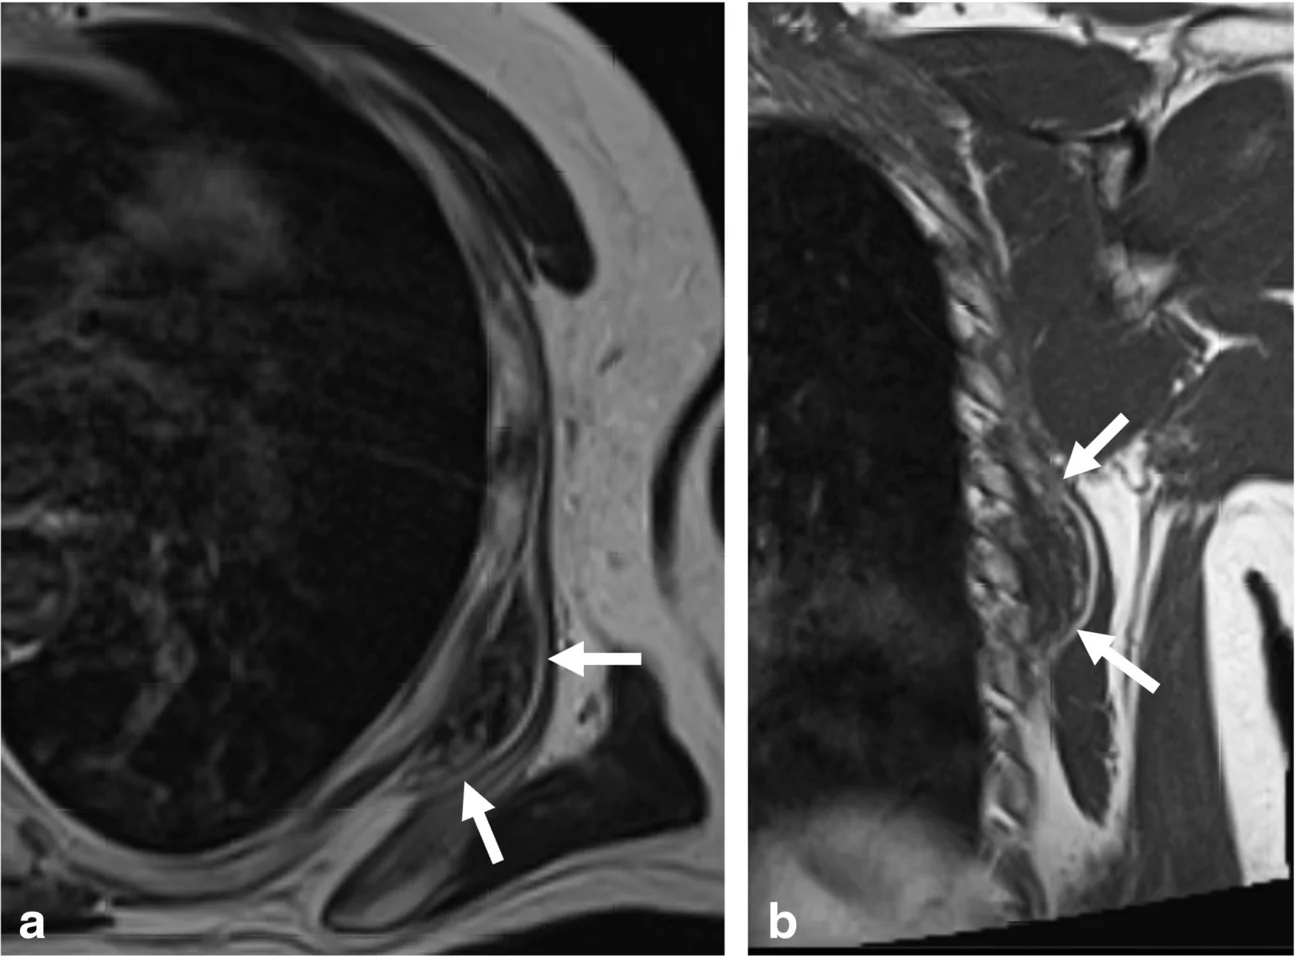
66-year-old man with symptomatic left elastofibroma dorsi. a Axial T2-weighted MR image (TE = 108 ms; TR = 4200 ms) shows a poorly circumscribed infrascapular soft-tissue mass with alternating fibrous low-signal and fatty high-signal streaks (arrows). b Coronal T1-weighted MR image (TE = 9.6 ms; TR = 375 ms) demonstrates the lesion interposed between the thoracic wall and adjacent scapulothoracic musculature (arrows)
